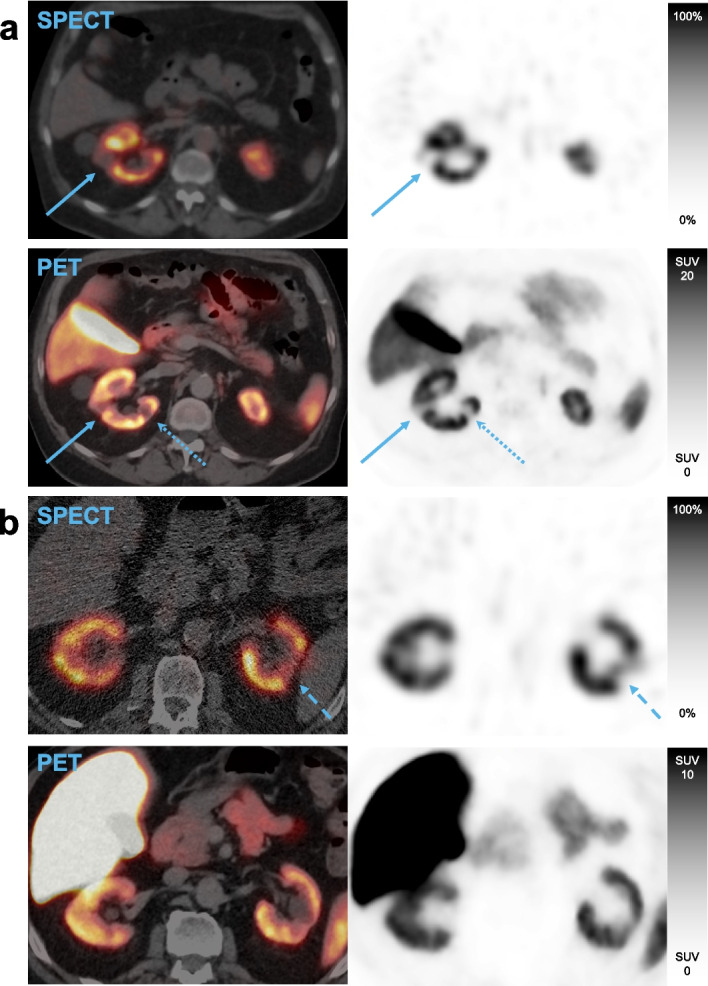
Example PET and SPECT images from two patients. Solid arrows in a indicate a defect found by readers on both PET and SPECT. Dotted arrows in a indicate a defect found on PET but not on SPECT. Dashed arrows in b indicate a defect readers found on SPECT but not on PET images. *SPECT*: single photon emission computed tomography; *PET*: positron emission tomography
